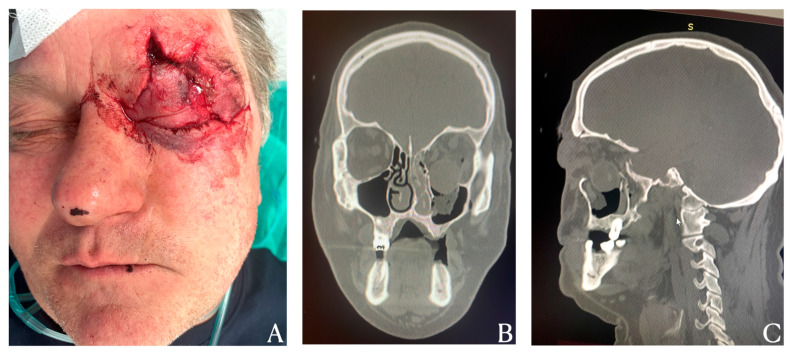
A 56-year-old male patient was admitted to the Department of Cranio-Maxillofacial Surgery at the University Hospital in Kraków on an emergency basis following blunt facial trauma as shown in Figure 1. Clinical examination revealed a displaced blowout fracture of the left orbital floor, accompanied by dislocation of the globe into the maxillary sinus and contused lacerations of the upper eyelid and frontal region. The injury occurred earlier that day when the patient was struck by a wooden block while operating a circular saw. Upon admission, the patient was fully conscious and able to recall the circumstances of the accident. Initial management at a district hospital included radiological assessment of the head and neck, ophthalmologic consultation, and the administration of tetanus prophylaxis. The patient was then transferred to a tertiary care center for specialized treatment. Upon examination, lacerations to the eyelid and frontal area were noted (A). Computed tomography (CT) scans in the coronal and sagittal planes revealed a complete fracture of the orbital floor, with the globe displaced downwards into the maxillary sinus and the extraocular muscles and optic nerve stretched (B,C) [1,2]. Orbital floor fractures most frequently result from blunt trauma to the periocular region and typically affect the orbital floor and medial wall, the most fragile components of the orbit. The injury mechanism generally involves either the transmission of force through the orbital rim or a sudden increase in intraorbital pressure caused by displacement of the globe. Blowout fractures are often associated with additional maxillofacial fractures and periorbital soft-tissue injuries. The most common causes are similar to those seen in general maxillofacial trauma and include motor vehicle collisions, interpersonal violence, falls, sports-related injuries, firearm incidents, and occupational accidents [3]. While orbital floor fractures are relatively common, complete disruption of the orbital floor with herniation of the globe into the maxillary sinus is an extremely rare and severe injury. Such cases pose substantial diagnostic and surgical challenges, with a high risk of functional sequelae including diplopia, enophthalmos, and restricted extraocular movement, as well as significant aesthetic deformity [4]. A comprehensive CT evaluation, including coronal, sagittal, and three-dimensional reconstructions, is essential for accurately assessing the extent of bony disruption and soft-tissue involvement. Particular attention should be given to the condition of the extraocular muscles and optic nerve, as visualization of these structures is critical for surgical planning and prognostic assessment.
